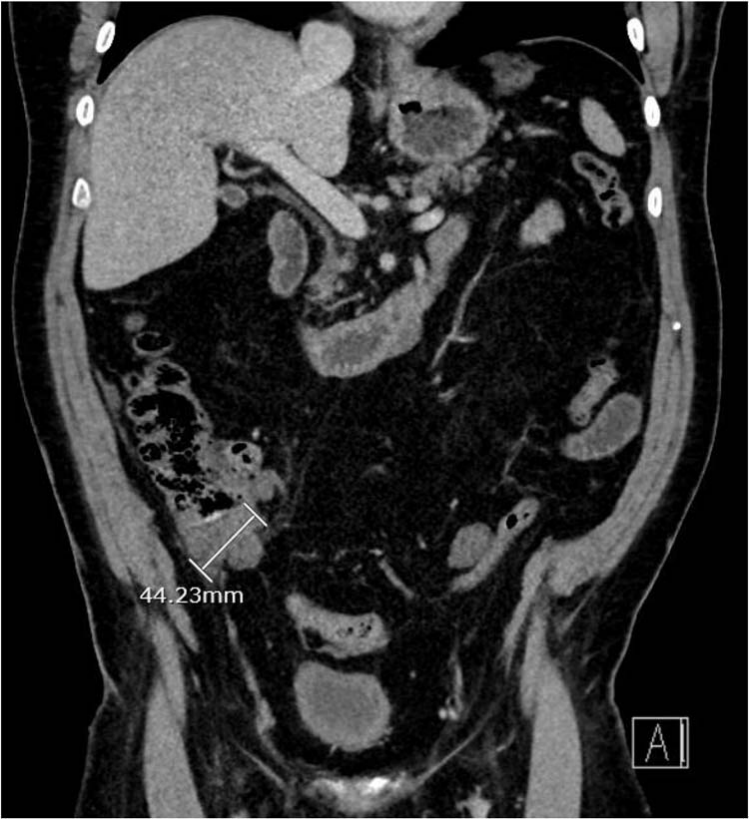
Three-month post-chemotherapy coronal CT abdomen and pelvis with contrast demonstrating a reduction in size of the primary appendiceal mass to 44.23 mm.
